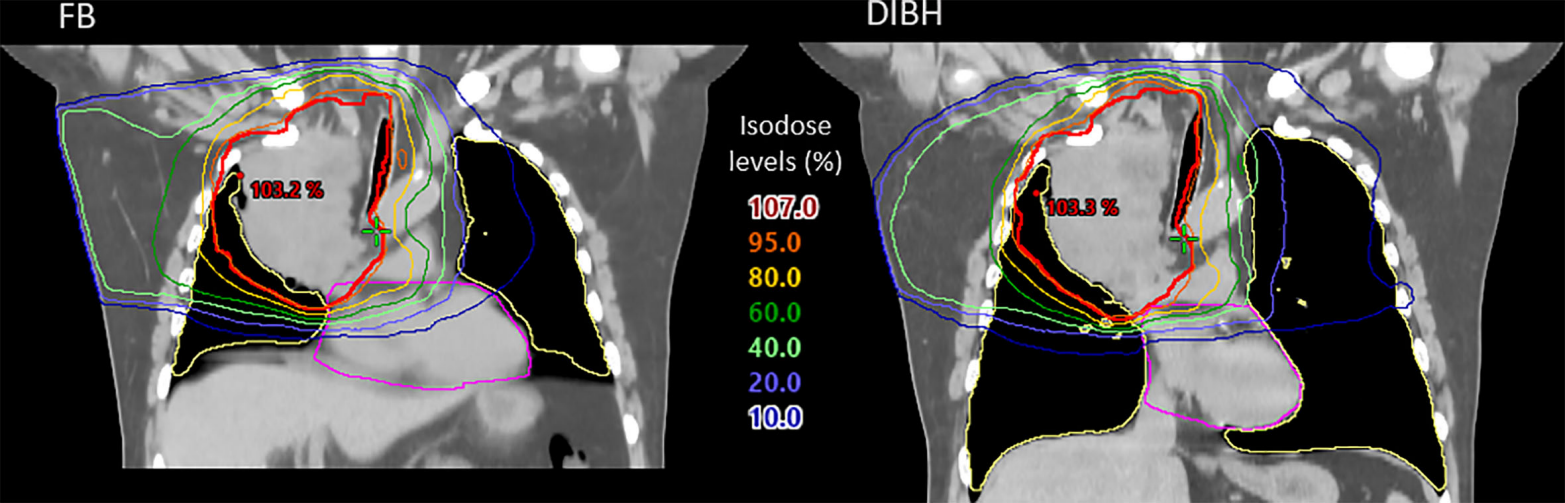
Dose distributions superimposed on planning CT scans of patient 1, showing enhanced sparing of OARs in DIBH (right) compared to FB (left). Contours are shown for the PTV (red), lungs (yellow) and heart (magenta). Isodoses are shown in percentage of the prescribed dose (60 Gy).
